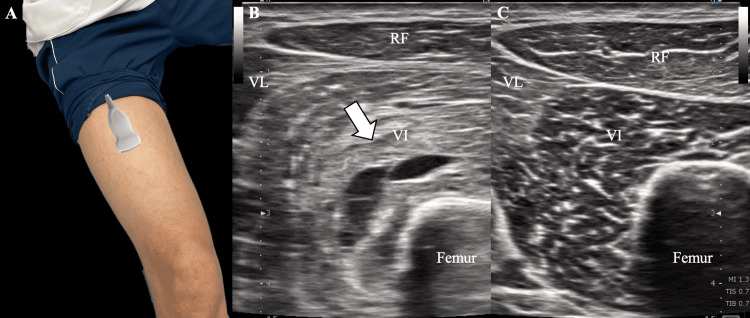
Ultrasound image of the thigh at initial presentation (A) The ultrasound probe was placed on the thigh as shown, and the obtained images are presented as ultrasound image of the thigh on the (B) affected side and (C) unaffected side Arrow: Hyperechoic and hypoechoic changes observed in the vastus intermedius. The hyperechoic changes were suggestive of tissue hardening, such as fibrosis associated with muscle injury, while the hypoechoic areas indicated possible hematoma or fluid collection RF: rectus femoris; VL: vastus lateralis; VI: vastus intermedius Image credit: The image was created and modified by the author Akira Ogawa (demonstration with a model)
